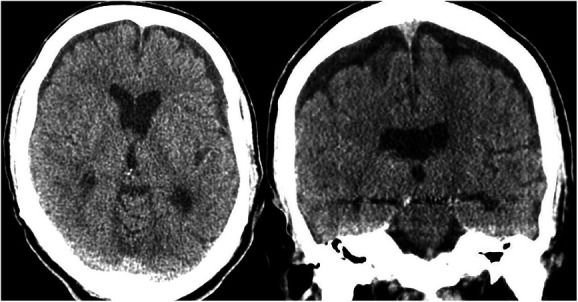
Computed tomography scan of a bilateral subdural hygroma in an axial and coronal plane.
